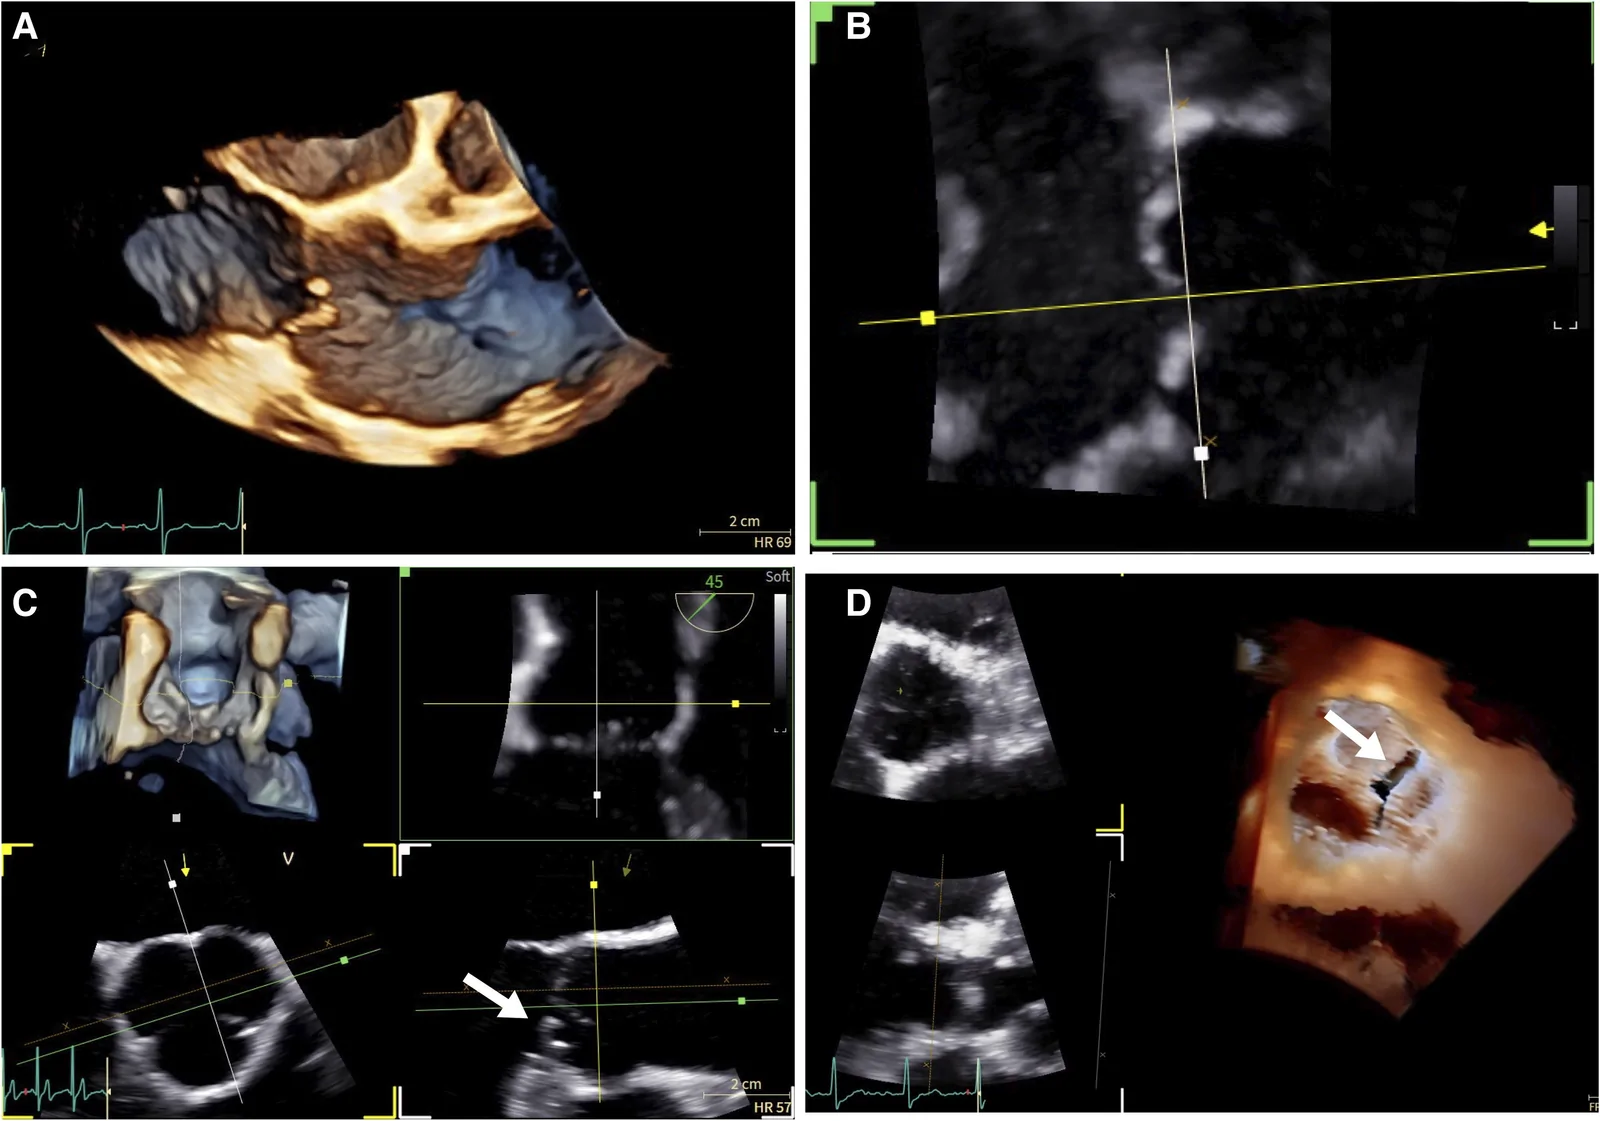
Mechanisms of aortic regurgitation (AR) according to El Khoury’s classification. Panel *A*: 3D ‘long-axis’ view of a patient with type I AR with dilatation of the VBR, aortic root, sinotubular junction, and tubular ascending aortic aneurysm. Panel *B*: Multiplanar reconstruction (MPR) of the same patients showing reduced coaptation height and symmetrical reduction in effective height. Panel *C*: MPR of a patient with complete prolapse of the right cusp (white arrow) in a tricuspid valve. Note that the effective height is < 0 mm. Panel *D*: 3D ‘en face’ view with transillumination modality obtained during a transthoracic exam. The light in aorta highlights the retraction of the left cusp (white arrow).
